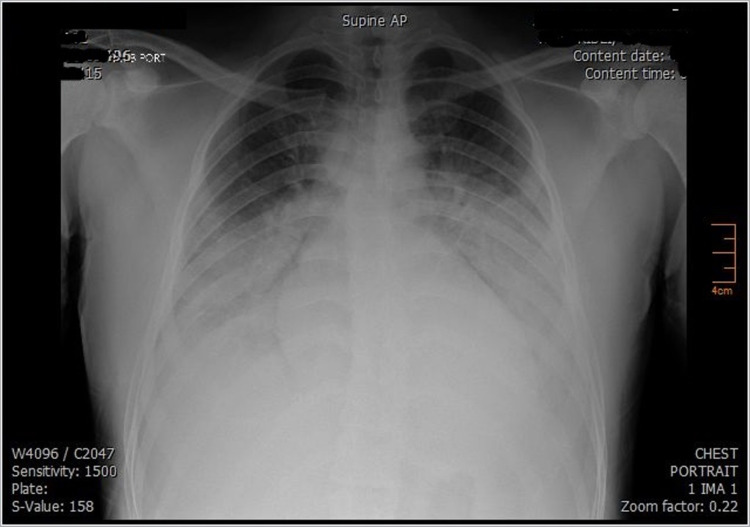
The second chest X-ray (CXR) of Mr. R. Second chest X-ray of Mr. R which was done after his health deteriorated. It showed bilateral pleural effusion and lung congestion suggestive of pulmonary edema.
